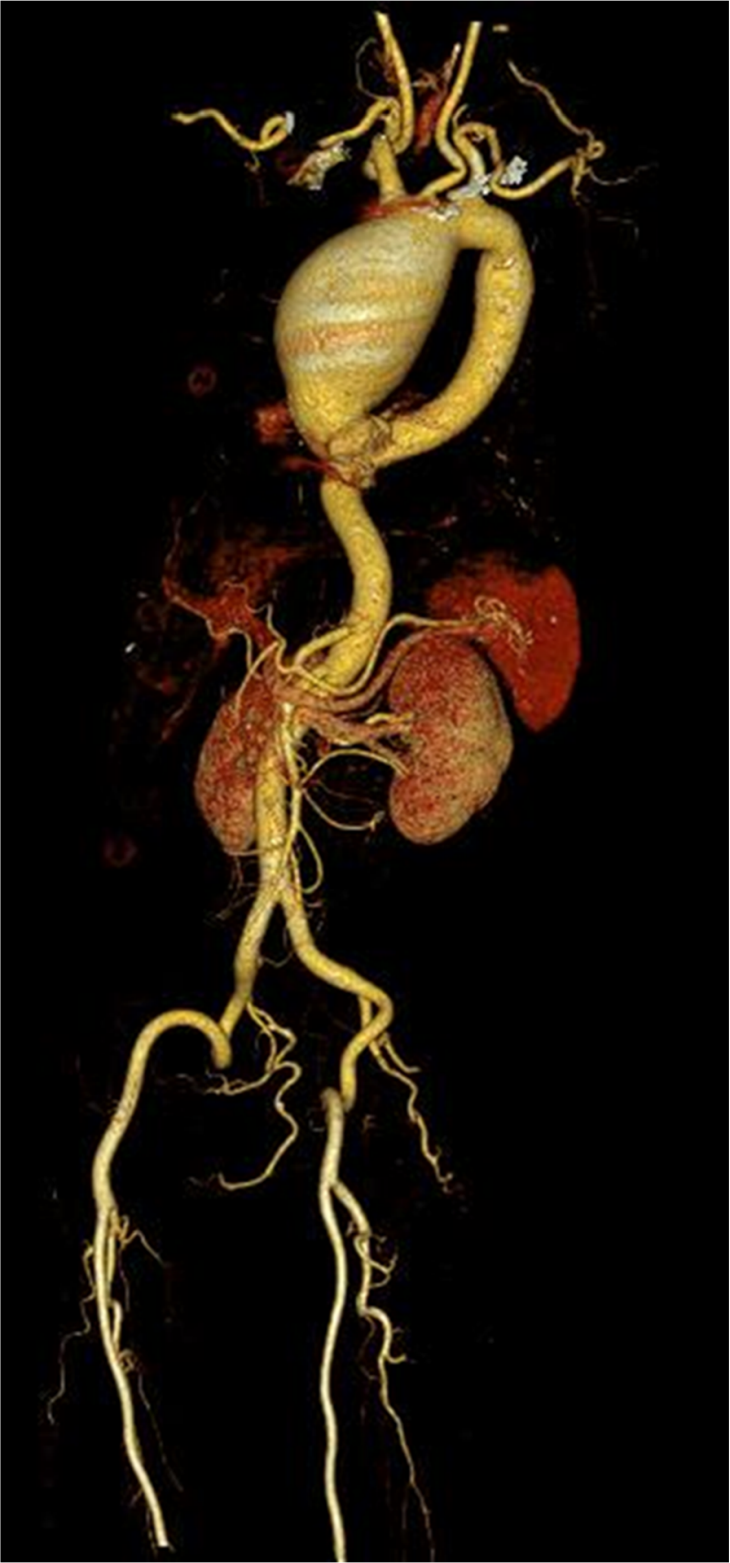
Computed tomography showing a giant ascending aortic aneurysm extending but confined to the proximal aortic arch
